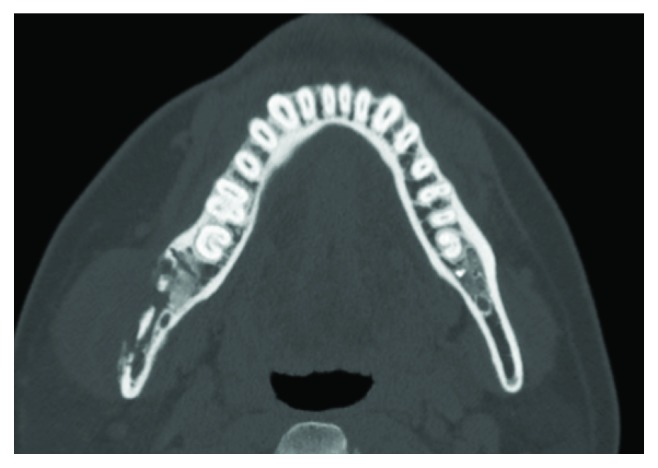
Coronal computed tomography (CT) scans obtained during the initial visit. CT shows a destruction of buccal cortical bone from the socket to the right mandibular angle and a formation of bone sequestrum. Although the lingual cortical bone is intact, there is periosteal reaction around the buccal bone destruction.
